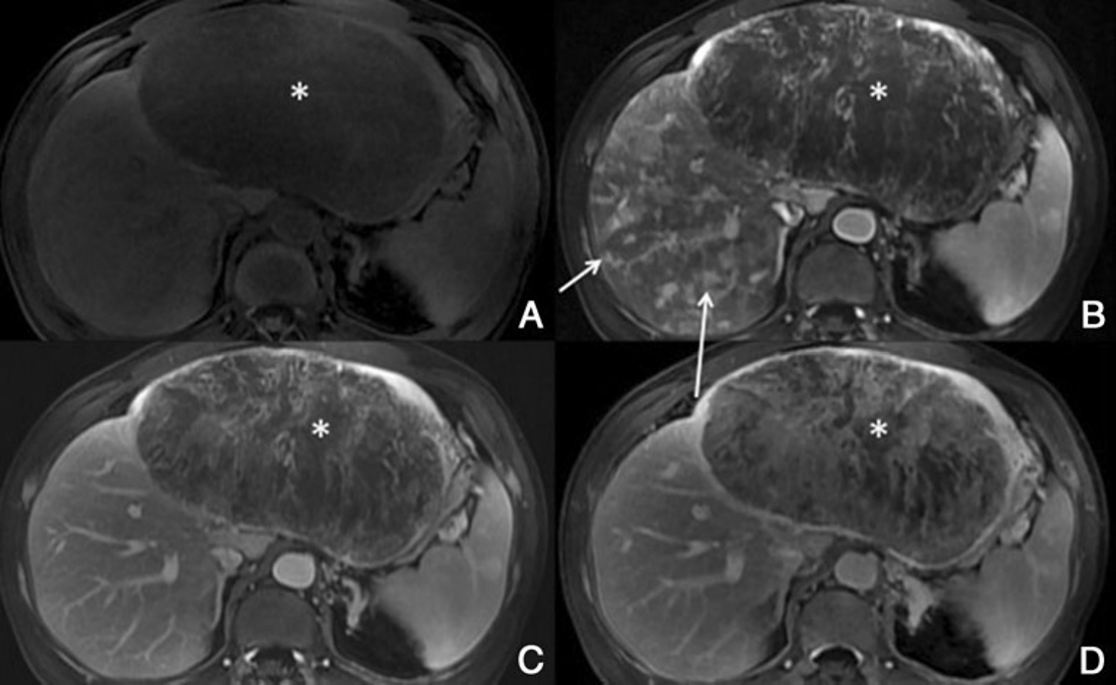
Multiple THIDs and a big HCC in a 47-year-old man with chronic liver disease. a Pre-contrast MRI, b arterial phase, c PV phase, d delayed phase. Big nodular lesion in the left lobe hypointense in pre-contrast imaging, with arterial enhancement, internal arteries, washout in the PV phase and heterogeneous uptake of Gd-EOB-DTPA in the HCP (*). There also seen multiple nodulariform hyperintense areas in the arterial phase (*arrows*), not seen in the other sequences—THIDs
